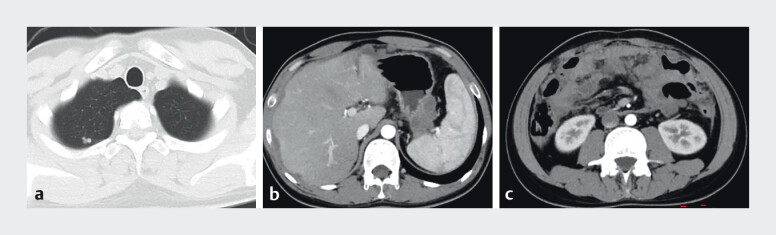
Contrast-enhanced CT revealed a right upper lung lobe nodule (
